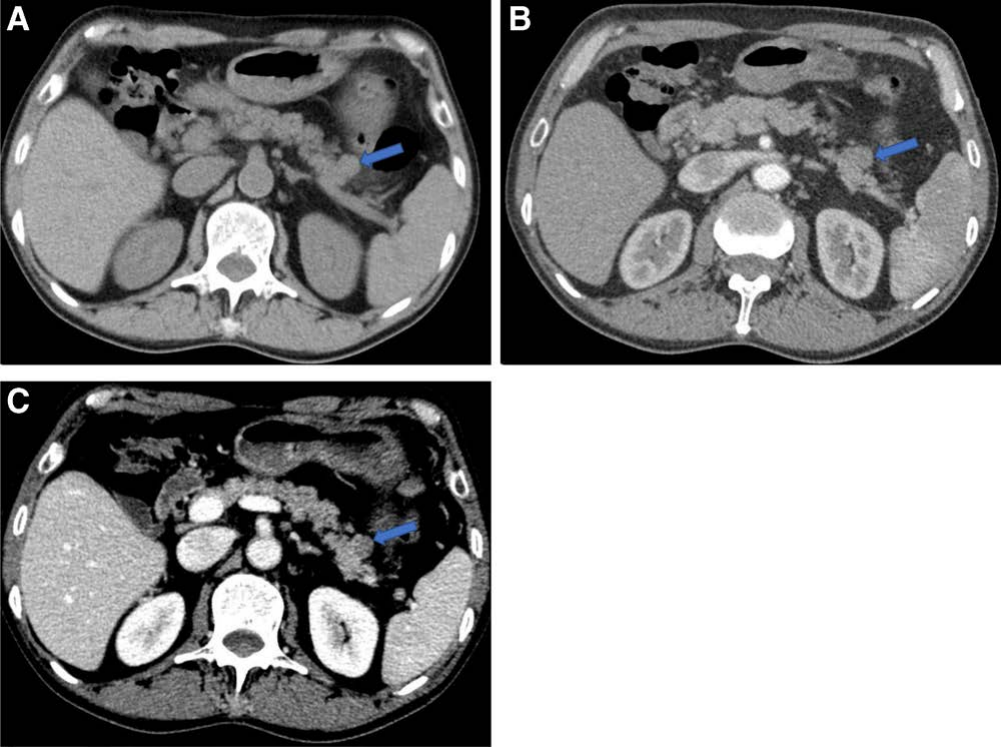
May 24, 2021 (14+ months after lung cancer surgery) enhanced abdominal CT examination. Plain scan (A) showed first order density nodule (blue arrow) in the tail of pancreas; arterial and venous phases (B, C) showed slight enhancement of the nodule of the tail of the pancreas, which was weaker than that of the adjacent pancreatic parenchyma, and the boundary was clearer than that of the plain scan. CT = computed tomography.
